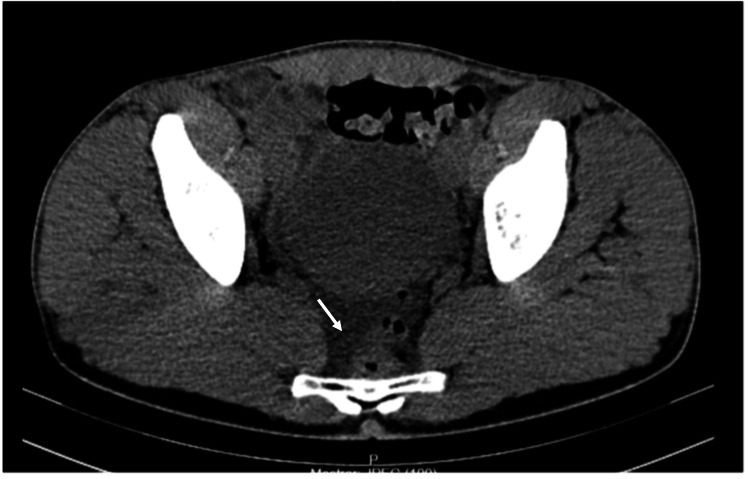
Abdominal computed tomography scan Small amount of intraperitoneal fluid with no visualization of the ileocecal appendix (arrow)
